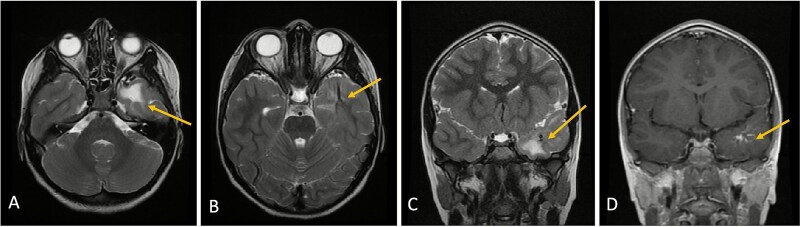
Axial lower cut (A)/axial higher cut (B)/coronal (C) T2-weighted MRI of the brain shows stable findings of a prominent area of T2 hyperintensity in the white matter of the left anterior temporal lobe, adjacent to the known DVA (arrow), which is clearly delineated on the contrasted sequence (D).
